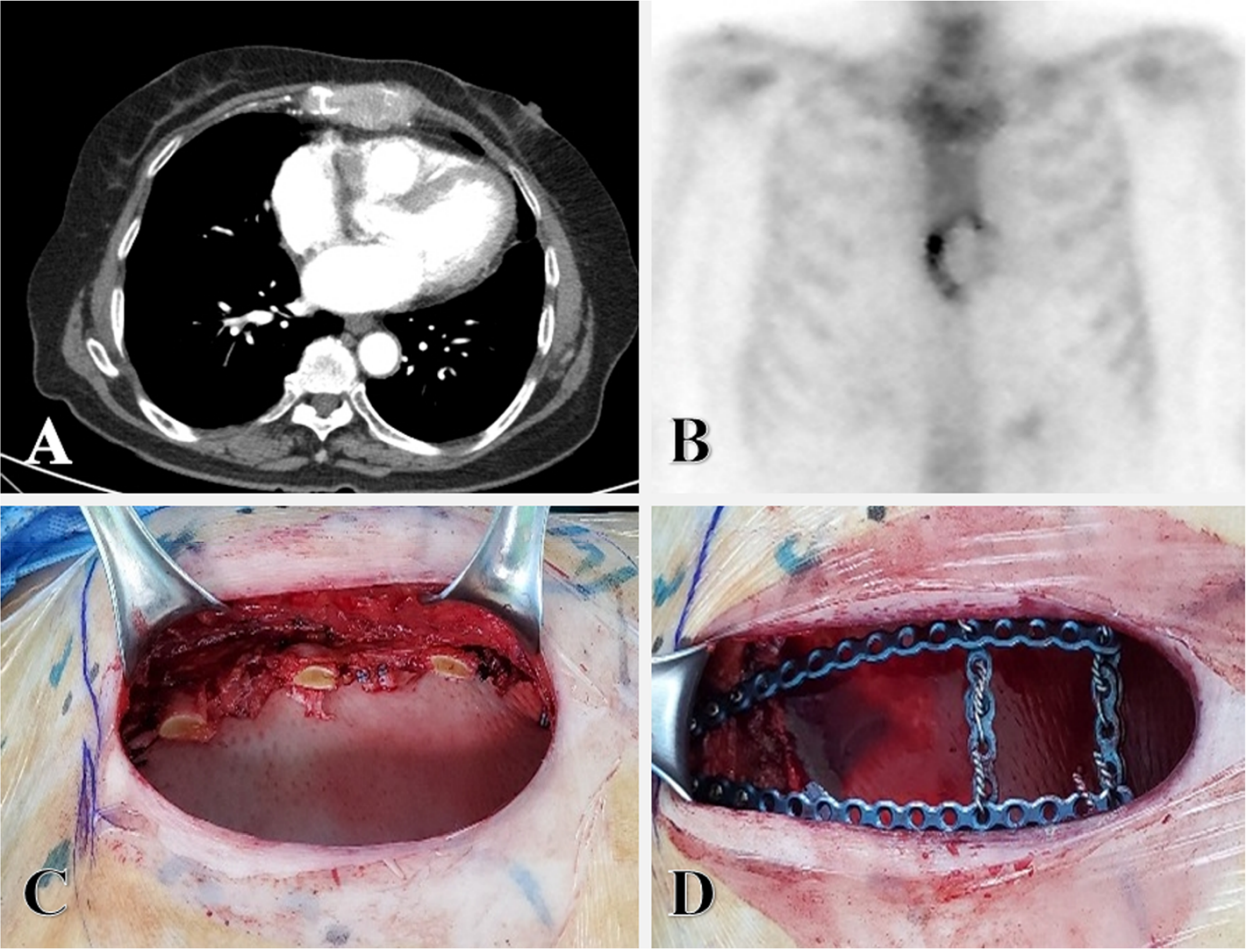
a Preoperative chest computed tomography and (b) whole body bone scintigraphy showing the tumor in the lower sternum. Intraoperative view of the anterior chest wall reconstruction with (c) acellular dermal matrix and (d) titanium plates
